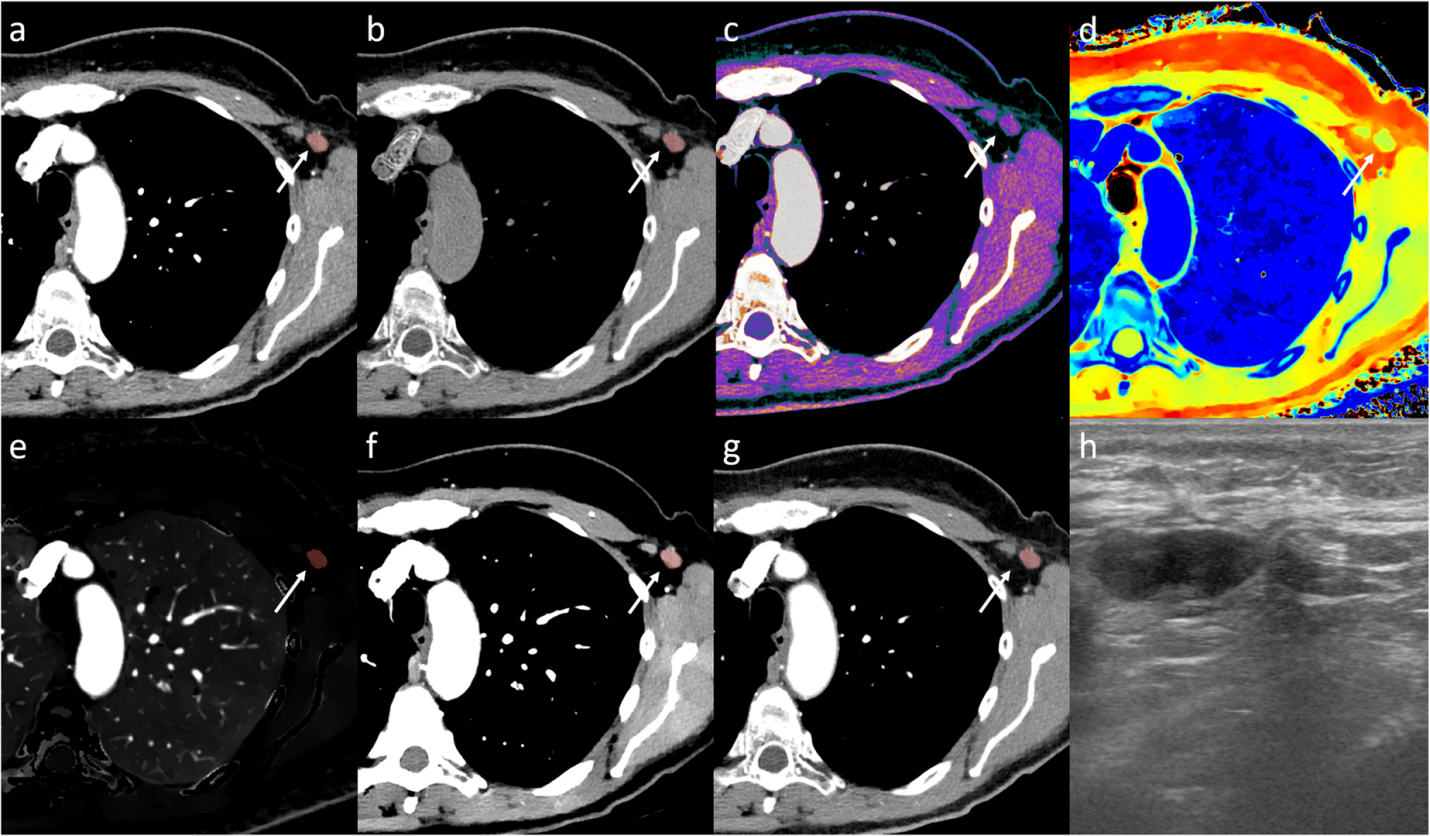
Arterial phase dual-layer spectral CT (DLSCT) examination of a woman with left-sided breast cancer and a lymph node in the left axilla. The lymph node does not show increased conventional CT HU (a), Δ contrast enhancement (c) (conventional CT (HU) (a) minus virtual non-contrast (HU) (b)), iodine density (e), or spectral slope ((mono-energy 40 keV (f) minus mono-energy 100 keV (g))/60 keV). However, *Z* effective (d) is decreased. Based on the cut-off values, both Δ contrast enhancement and conventional CT HU diagnosed the lymph node as non-metastatic. Axillary ultrasonography (h) described the lymph node as suspicious, and fine-needle aspiration contained malignant tumour cells. Sentinel lymph node biopsy contained malignant tumour cells from invasive ductal carcinoma
